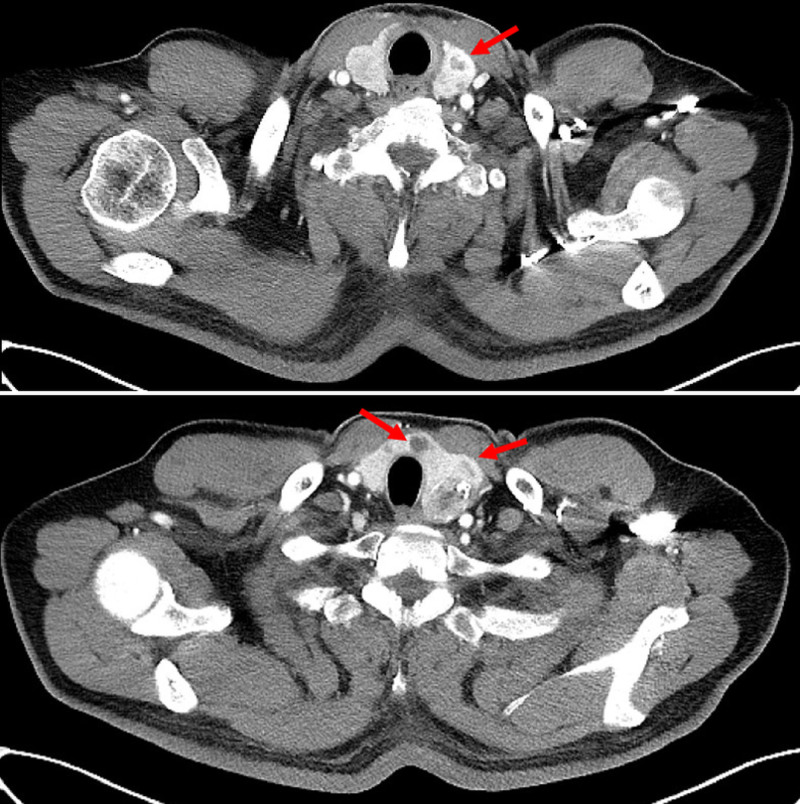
Multiple thyroid nodules were found in chest CT during staging workup of gastric cancer.
